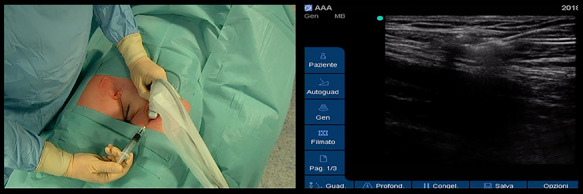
Bilateral injection of the ICG in the inguinal nodes with an ultrasound guide.
